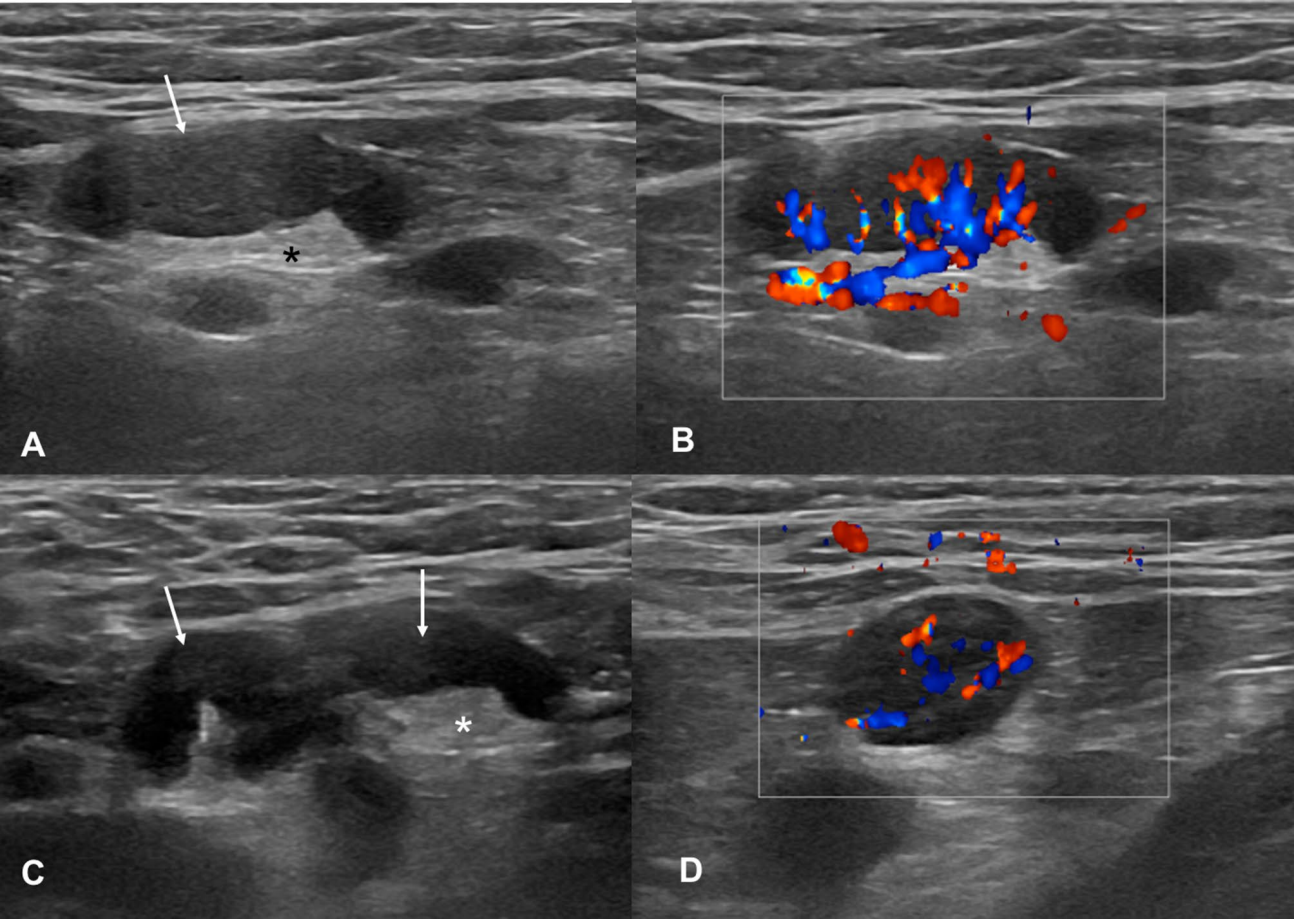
B-Mode (A, C) and color-Doppler (B, D) US examination performed 3 days after receiving the second dose of vaccine. Enlarged (maximum diameter 21 × 7 mm) axillary lymph node with preserved fatty hilum (* in A and C), diffuse cortical thickening (6 mm, arrows in A and C) and vivid hilar and cortical vascularization (B, D) were found
